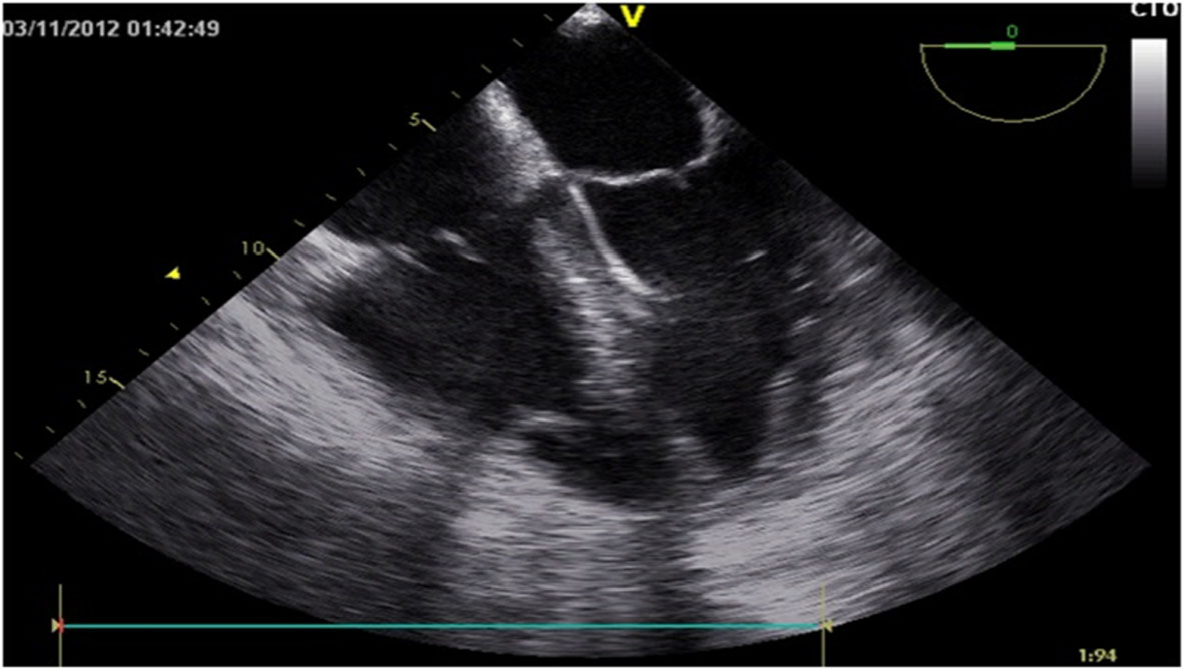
Transesophageal echocardiography in the 3rd post-operative day, showing unloaded Left ventricle after Impella 5.0 repositioning and unloaded right ventricle by VP-ECMO
